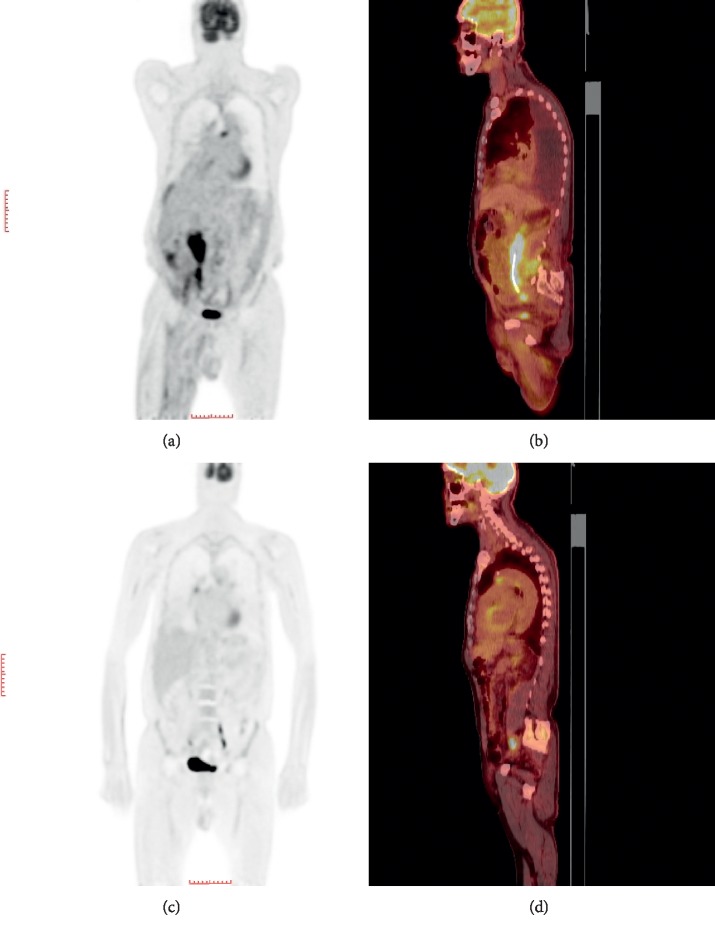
Initial diagnostic PET-CT scan with soft tissue mass encasing the right proximal ureter (a and b). Complete resolution of right periureteral mass on treatment completion PET-CT scan (c and d).
